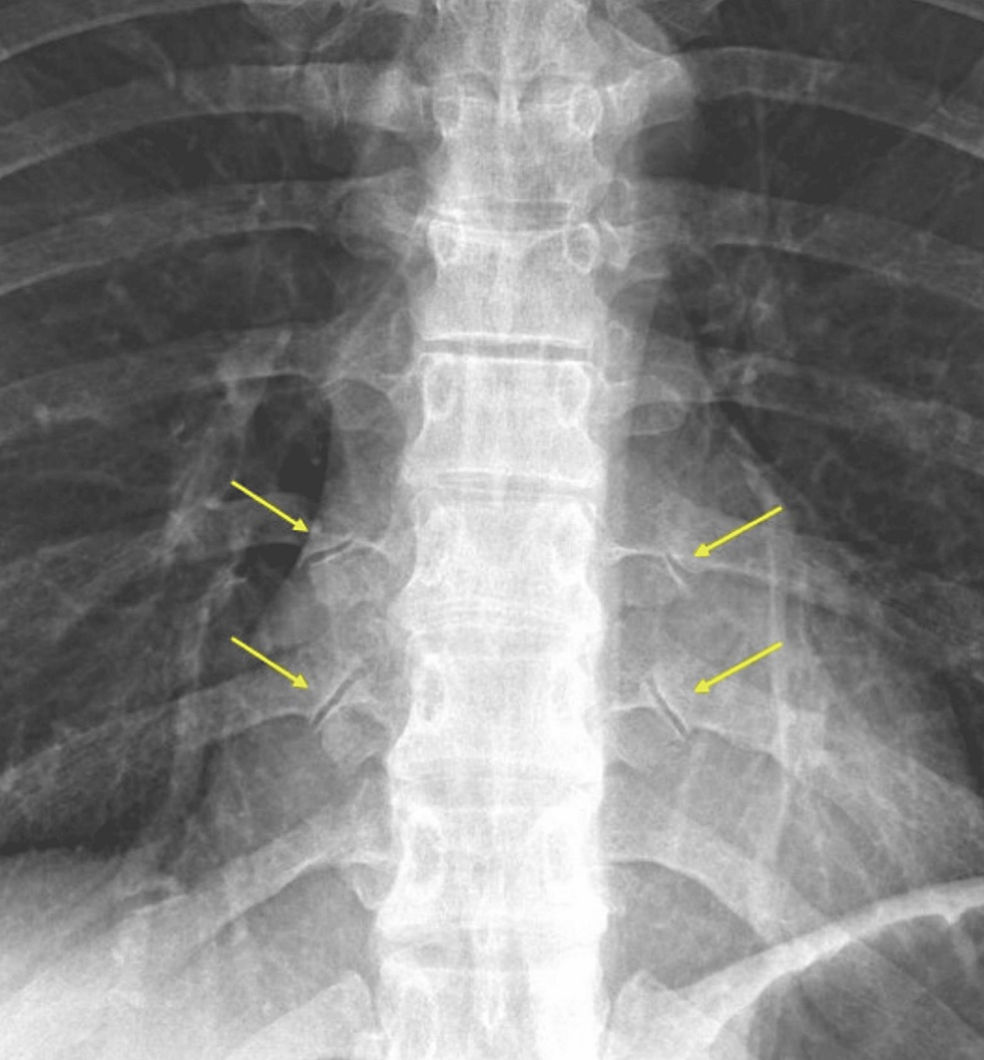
X-ray of the thoracic spine showing degenerative changes in the bilateral T8 and T9 costotransverse joints (yellow arrows).
